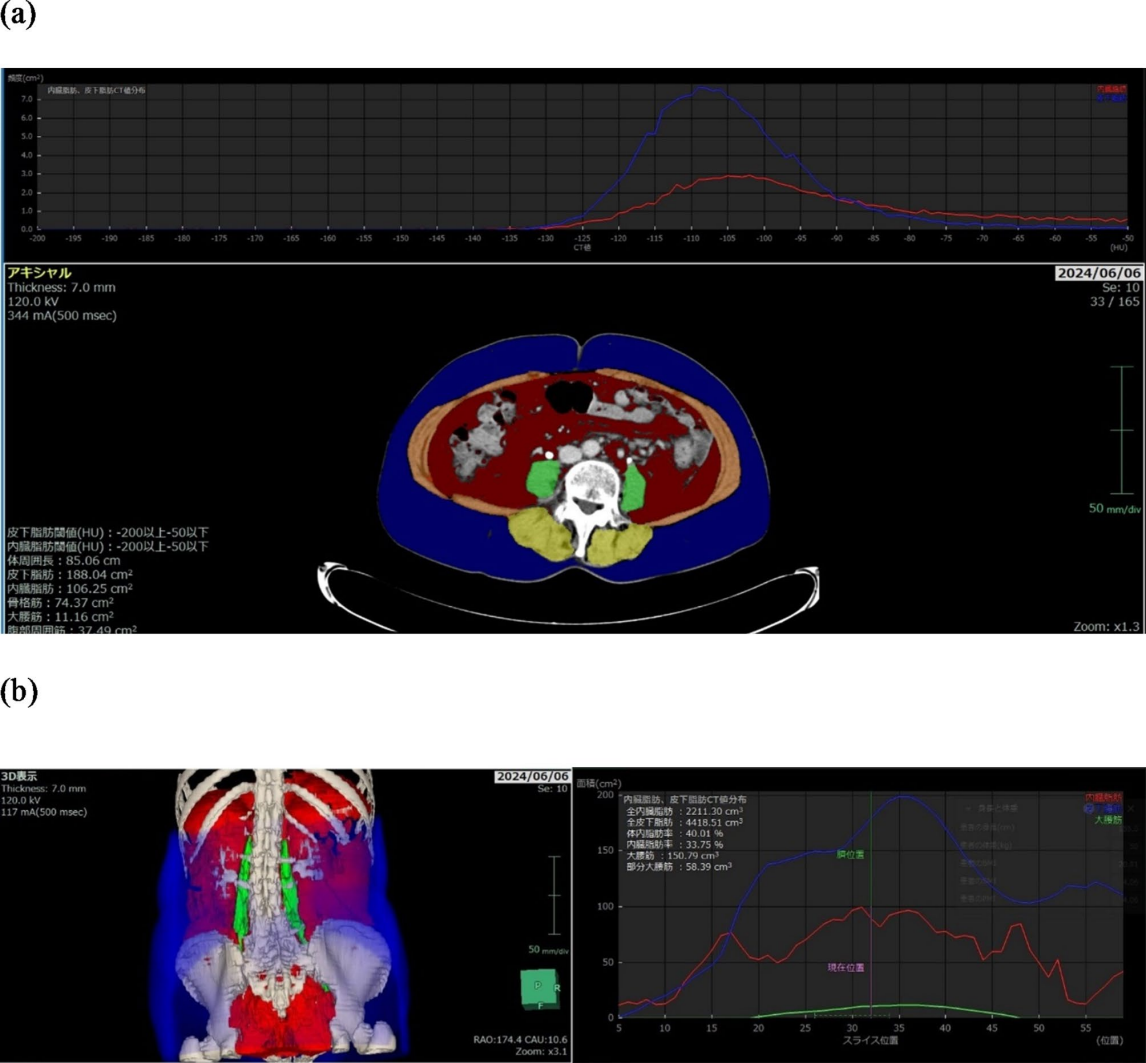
Representative images of body composition components were reconstructed using the SYNAPSE VINCENT 3-dimensional medical image analysis system. (a) Cross-sectional computed tomography image at the level of the umbilicus. Areas colored in yellow and orange indicate the skeletal muscle area, and those colored green indicate the psoas muscle area. (b) Three-dimensional image construction. Green areas indicate the total volume of the psoas muscle.
